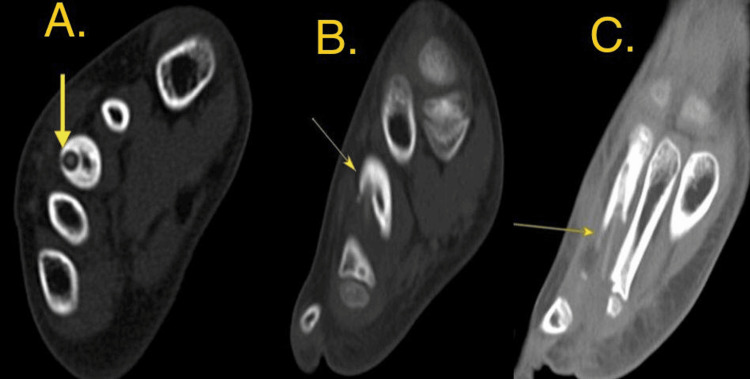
CT image: Slice thickness and reconstruction (0.8 mm) The long faintly dense structure (foreign body) density is around (150 HU) seen partially emerging into and surrounded by dense cortex with consequent narrowing of the medullary canal while its distal portion is seen extra osseous. (A: axial view, B: sagittal view & C: coronal view) Chronic medullary sclerosis & tunneling harboring a relatively dense, elongated foreign body (arrow) within the medullary canal of the third metatarsal, with associated bone destruction.
